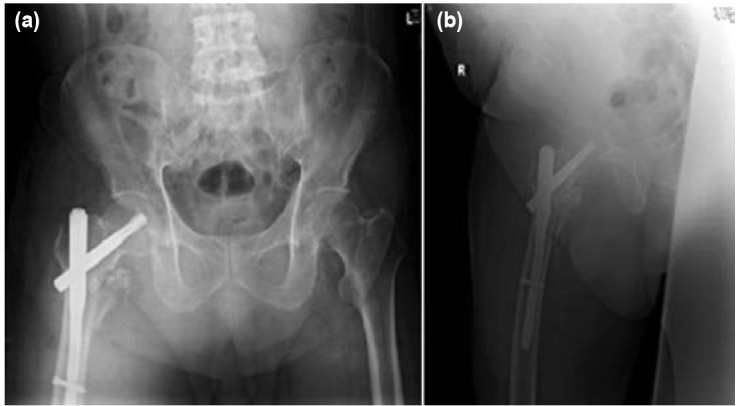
(a) Anterior-posterior view and (b) Lateral view of post-operative radiographs showing satisfactory reduction and fixation of fracture.
